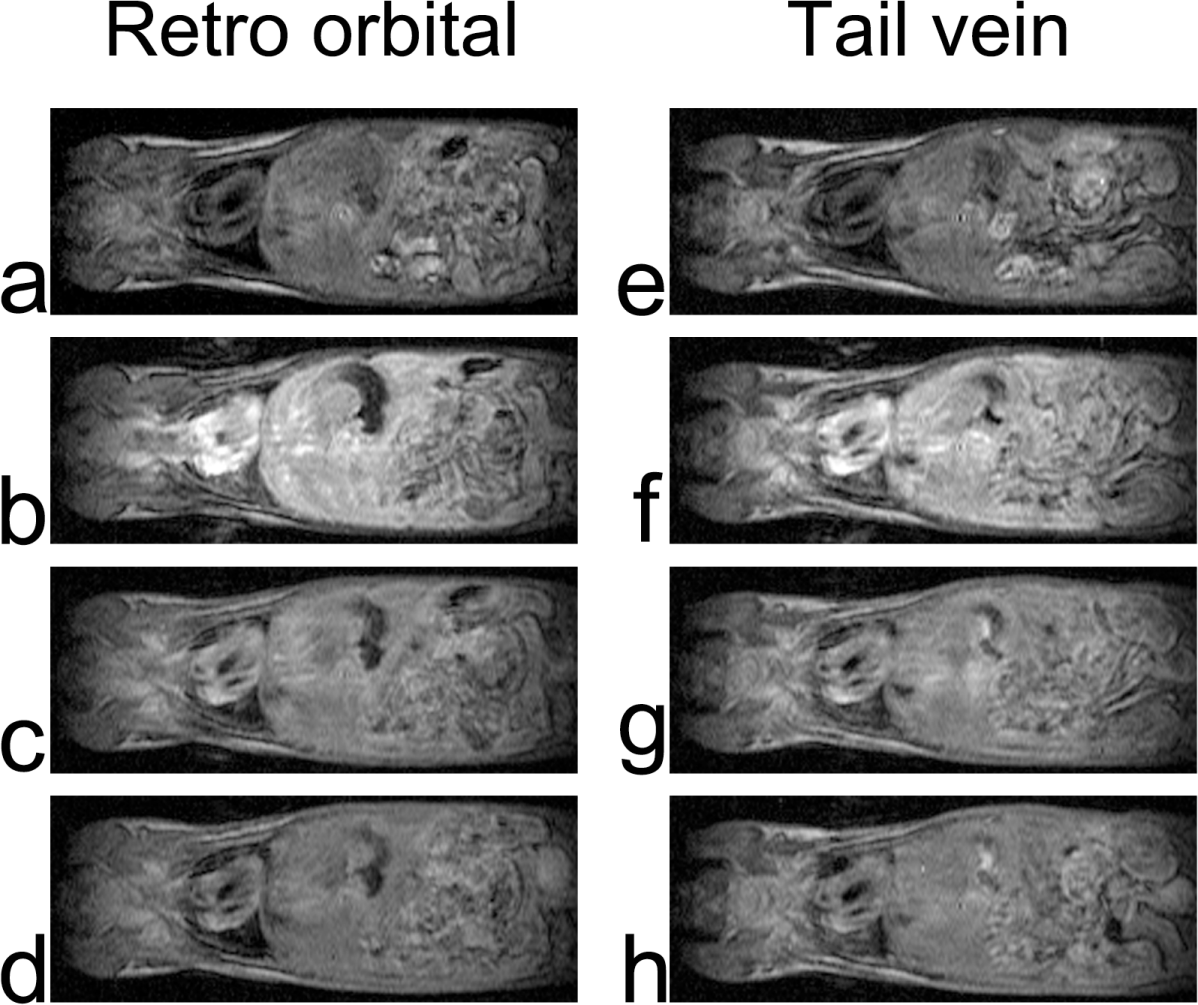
Typical thoracoabdominal coronal MRI. Left column, from top to bottom, images acquired before (a), 30 s (b), 3 min 30 s (c), and 10 min (d) after delivery of the contrast agent via the retro-orbital route. Right column, images obtained before (e), 30 s (f), 3 min 30 s (g), and 10 min (h) after delivery of the contrast agent via the tail vein route.
